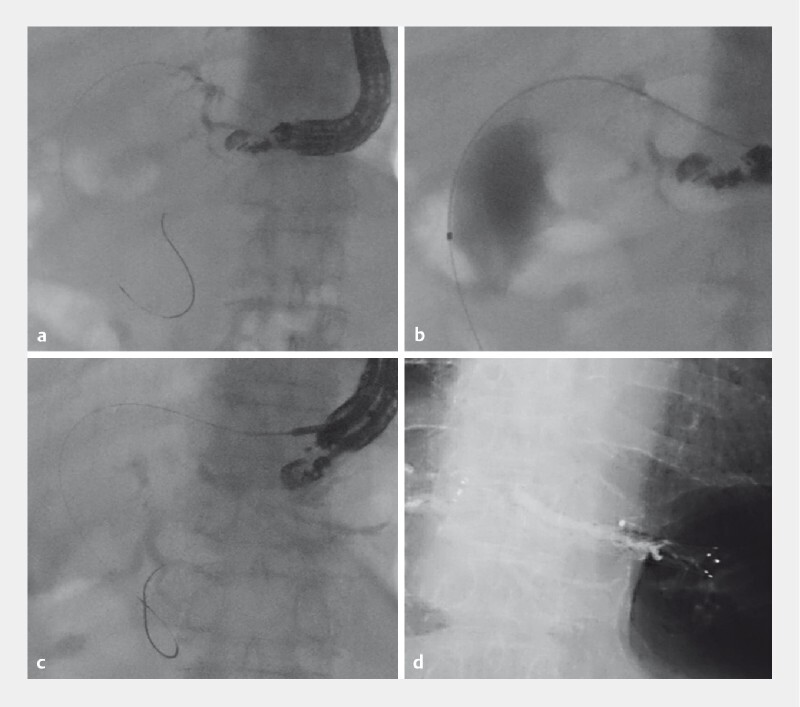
Preprocedure coronal computed tomography showing a dilated bile duct due to pancreatic head cancer (arrowhead).
